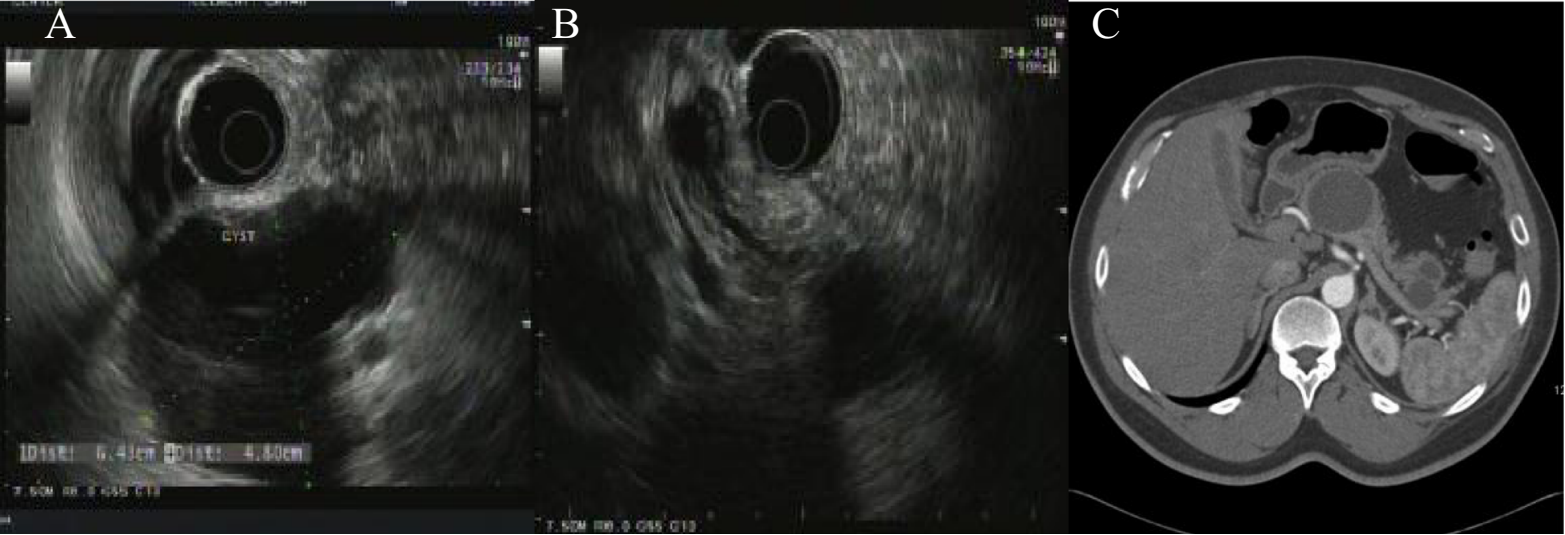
Endoscopic ultrasonography and computed tomography. A-B) Endoscopic ulrasonography showing cysts in neck, body and tail of Pancreas. C) Computed tomography of pancreas showing cystic mass with hemorrhage.
